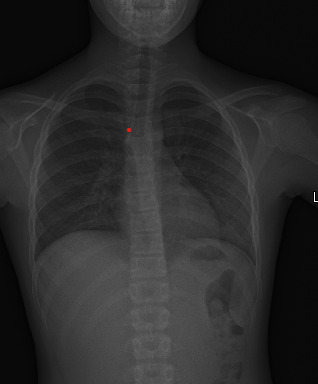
Antero-Posterior Chest X-ray showing bilateral subtle interstitial reticular pattern in the peripheral inferior pulmonary lobes and slightly increased peribronchovascular markings
